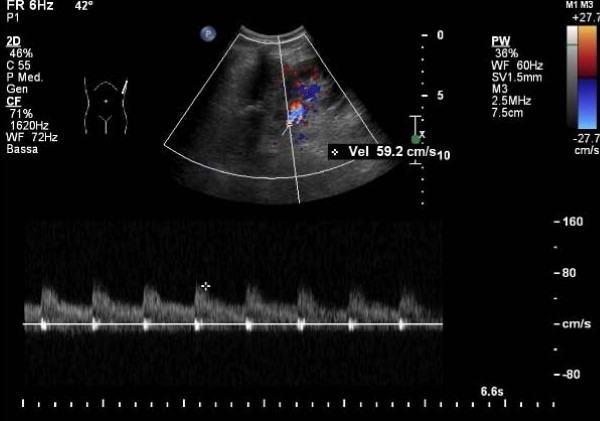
Spectral Doppler sonogram showing the arteriovenous malformation (AVM). The image aliasing area highlights the AVM.
